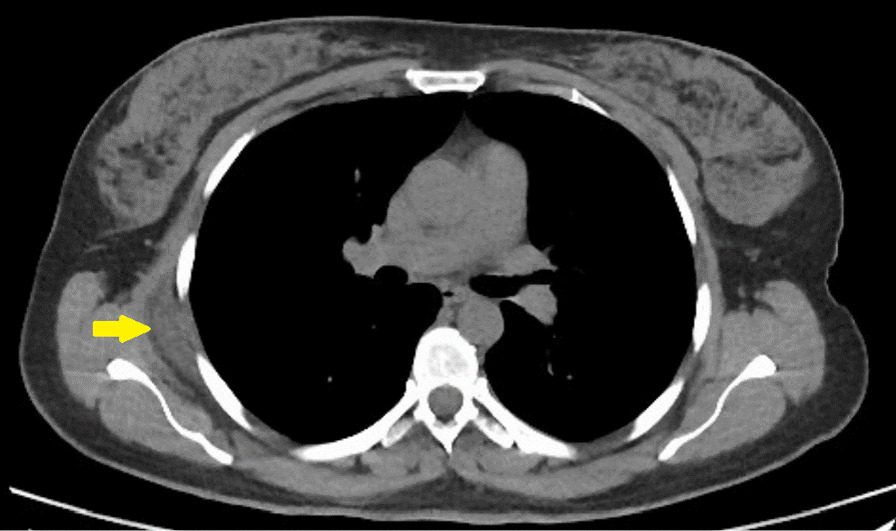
Computed tomography of a case with unilateral fibrolipoma (yellow indicator)
